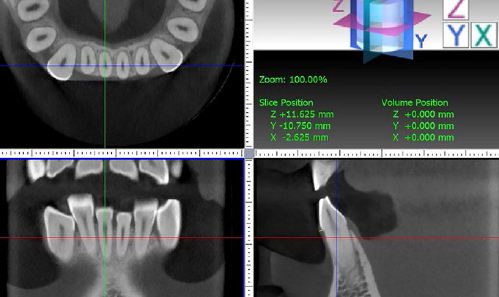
Measurement procedure in the CBCT images.
